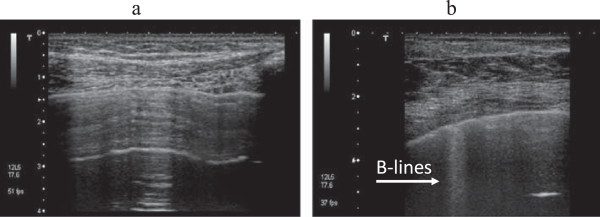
Standard ultrasound image of the lung in case of a healthy lung (a) and inpresence of sonographic interstitial syndrome (b).
